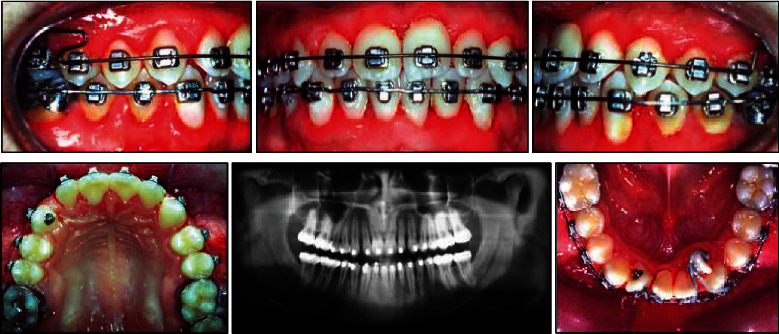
Leveling in both arches with space gaining with lateral incisor derotations in the lower arch along with panoramic X-ray before brace removal.
